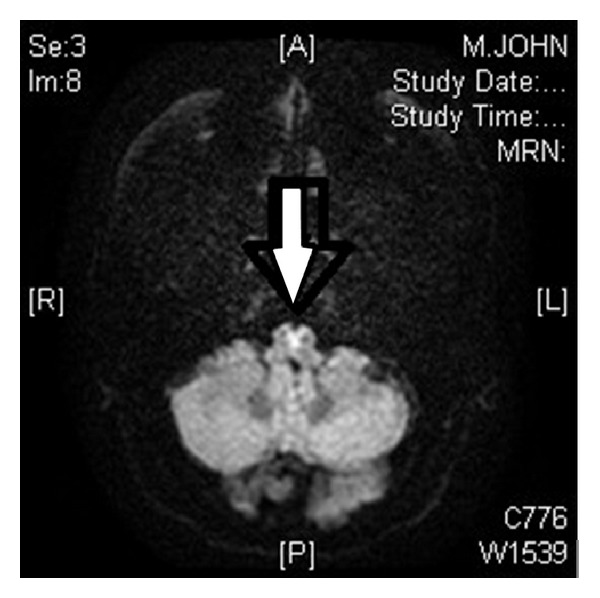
MRI of brain and DWI at presentation. Abnormal signal at DWI, a midline dot-linear signal, was similar to an artifact.
